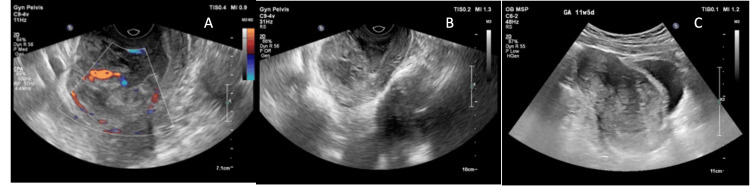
(A) Heterogenous lesion with enhanced vascularity on ultrasonography; (B) empty uterine cavity; (C) thinning of myometrial wall.
